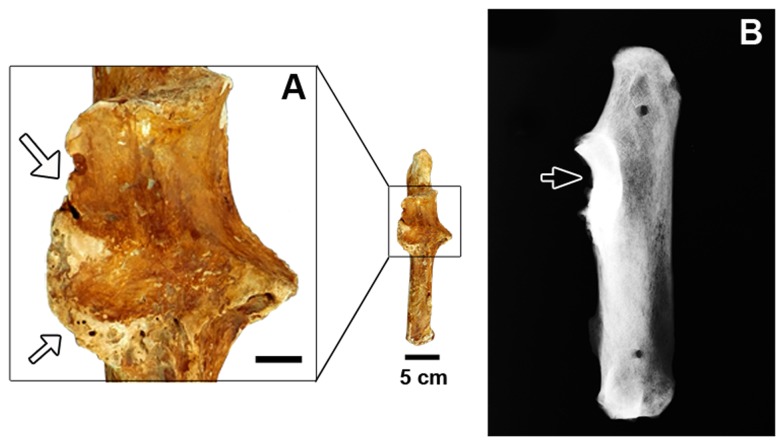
Right ulna of *Glyptotherium* (MCC 491-V). (A) A detail of the trochlear and radial notch in a cranial view; (B) Lateral radiography. The large white arrow indicate bone erosion, the smaller white arrow indicates bone growth and the black arrow indicates sclerosis. Scale bar in the detail A = 1 cm.
